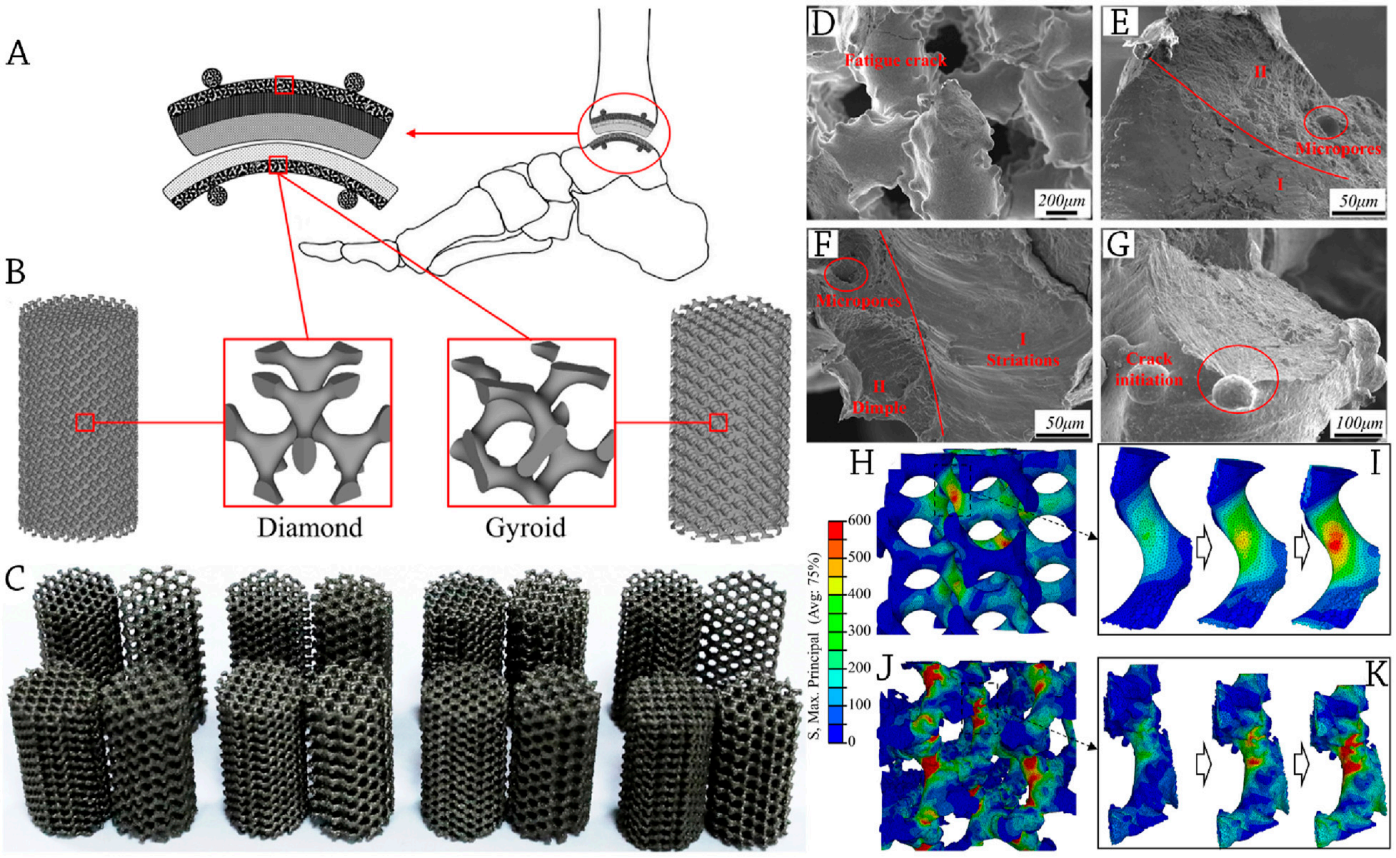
(A) A description of a prosthetic device for the foot and ankle that includes bionic porous scaffolds, which serve both biological and biomechanical purposes; (B) The prosthetic device incorporates specially designed porous scaffolds; (C) The scaffolds are created using Electron Beam Melting (EBM) and are tested for compression and fatigue resistance. The SEM was used to examine the fracture morphologies of the scaffold after fatigue testing; (D) Struts exhibiting fatigue fractures; (E, F) A close examination of the fracture section reveals two distinct morphologies. The first morphology, labelled as I, consists of striation areas that indicate the beginning and propagation of the crack. The second morphology, labelled as II, is characterized by dimple regions where the crack ultimately breaks. (G) Initiation of cracks from surface irregularities. (H–K) The stress distribution of the modelled scaffold is visualized using colored patterns in a FEA, highlighting the progression of maximum primary stress. The designed model exhibits a more homogeneous distribution of stress. The CT-reconstructed model reveals that stress is localized at the base of the uneven surface. Reprinted with permission from Liu et al., (2023).
